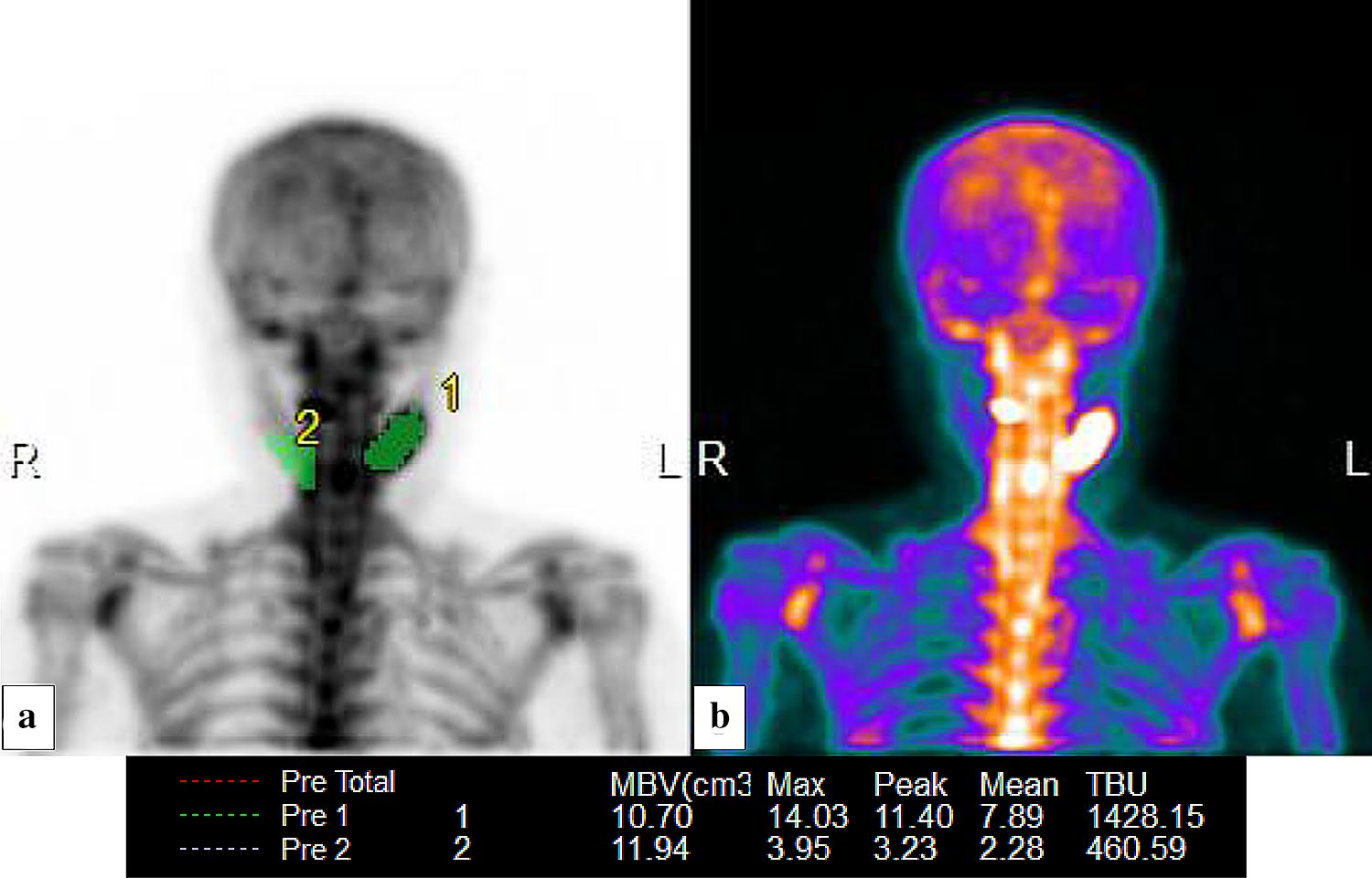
Volume of interest setting on the GI-BONE^®^ software. a Detection result of lesion with high accumulation and unaffected control region and b original image. The VOI of a lesion (1) in the left mandible was semi-automatically placed over a lesion with an area of high tracer accumulation using the threshold method available within the software. The threshold value was automatically calculated by obtaining a histogram from the target range and adding 0.5 standard deviation (SD) to the base value. This base value was obtained as the pixel value reduced from the most frequent value to 95%. The VOI in an unaffected area (2) was manually set within a mandibular bone region without tracer accumulation. MBV, SUV_max_, SUV_peak_, SUV_mean_, and TBU values were automatically calculated by the GI-BONE^®^ software. The calculated quantitative values are shown below the images. The SUV_peak_ value was not examined in this study. MBV, metabolic bone volume; SUV_max_, SUV_peak_ and SUV_mean_, maximum, peak, and mean standardized uptake values, respectively; *TBU* total bone uptake, *VOI* volume of interest
